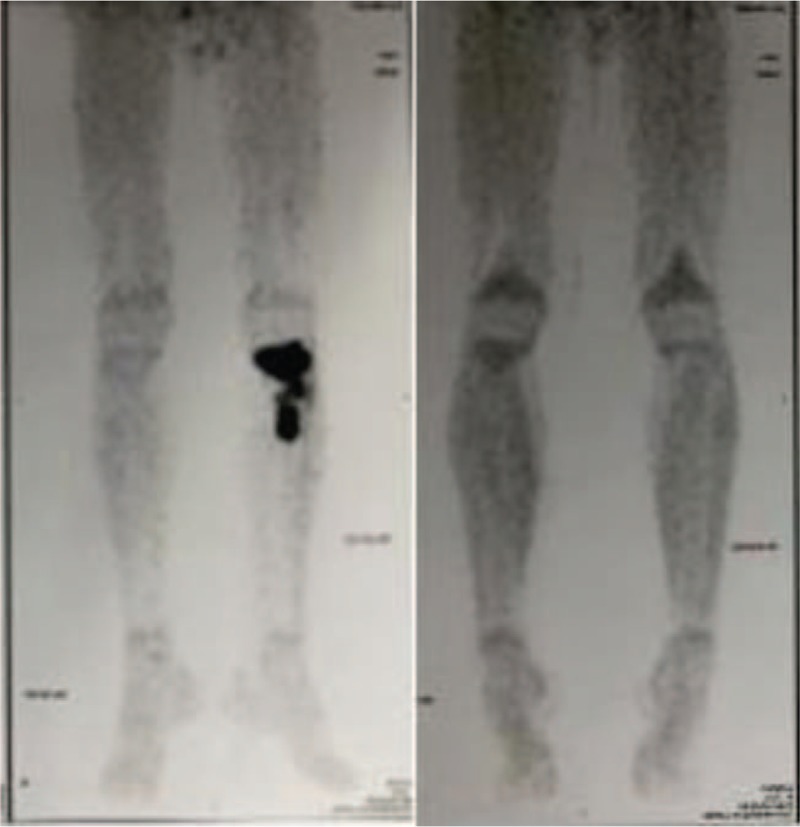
PET-CT before treatment (A) PET-CT after treatment (B). PET-CT = positron emission tomography/computed tomography.
